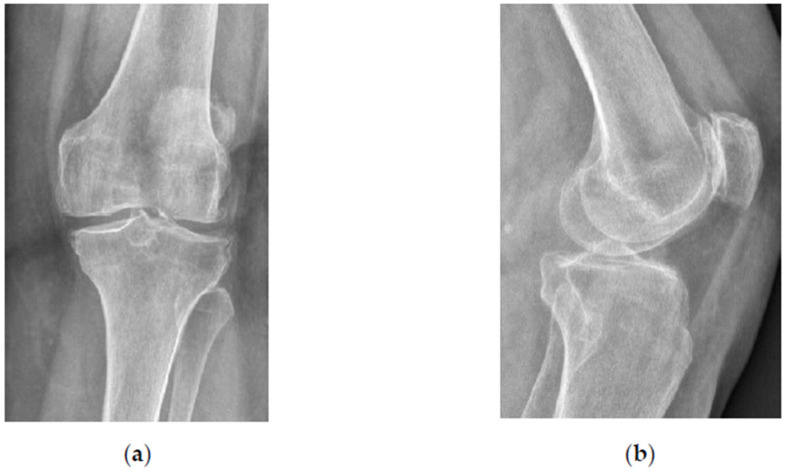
Standing X-ray images of the left knee. (a) Anteroposterior view; (b) lateral view.
